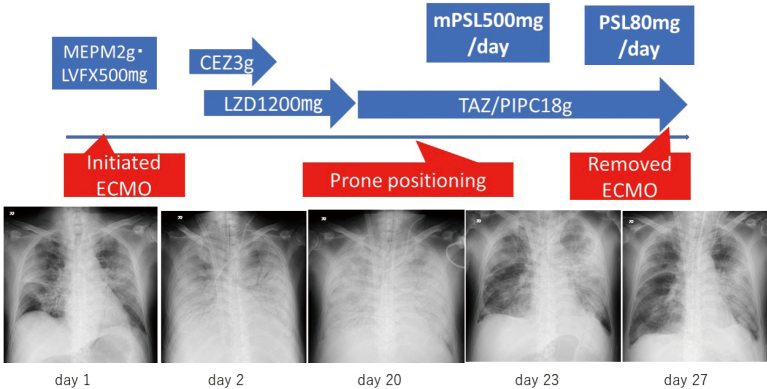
Chest X-ray images in time series of a 67-year old man during hospitalization for severe COVID-19-induced acute respiratory distress syndrome. Chest X-ray on day 1 shows bilateral basal consolidation with periphearal ground-glass opacity. Chest X-ray on day 2 shows bilateral pulmonary infiltrate mainly in hilar region. Chest X-ray on day 20 shows bilateral diffuse pulmonary infiltraes with air bronchogram. Chest X-ray on day 23 shows improved bilateral lung opacity (mainly in the right lobe of lungs). Chest X-ray on day 27 (extracorporeal membrane oxygenation removed).
